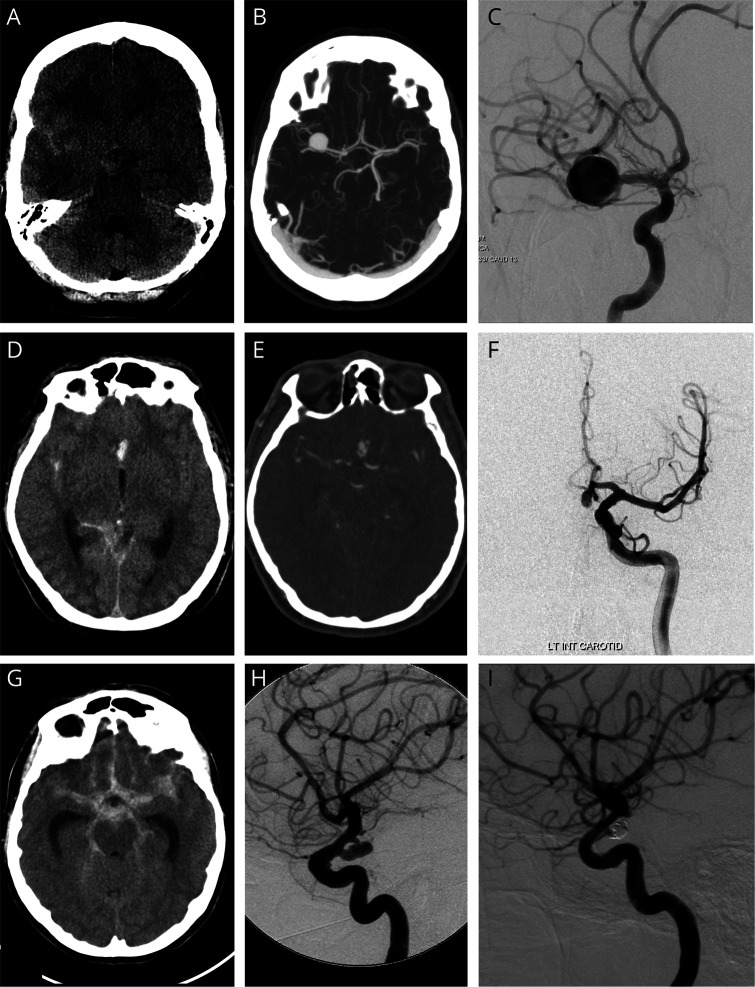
Familial Intracranial Aneurysm (IA) With ANGPTL6 Genetic Variants Had Typical History and Imaging of IA Patient 1: a 48-year-old woman presenting with subarachnoid hemorrhage (SAH) within the right sylvian fissure and suprasellar cistern on the axial noncontrast CT examination (A), from a large right terminal M1 segment right middle cerebral artery aneurysm demonstrated on a maximal intensity projection axial CT angiogram (B), and an oblique view of a selective injection into the right internal carotid artery on digital subtraction cerebral angiography (C). Patient 2: a 41-year-old man presenting with SAH within the right sylvian fissure, anterior interhemispheric fissure, and right quadrigeminal cistern on the axial noncontrast CT examination (D), from an A1–A2 junction aneurysm of the left anterior cerebral artery demonstrated on axial CT angiography (E), and a Towne projection from the selective injection of the left internal carotid artery on digital subtraction cerebral angiography (F). Patient 3: a 57-year-old woman presenting with extensive SAH within the sylvian fissures, anterior interhemispheric fissure, as well as the perimesencephalic and ambient cisterns on the axial noncontrast CT examination (G), and from a posterior communicating segment aneurysm of the left internal carotid artery demonstrated on the lateral projection from the selective injection of the left internal carotid artery on digital subtraction cerebral angiography pre-(H) and post- (I) coil embolization with satisfactory exclusion from the arterial circulation.
